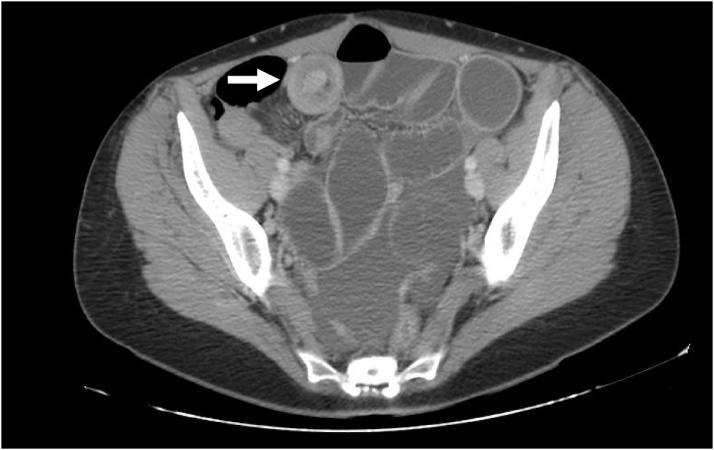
Abdominal CT evidenced a complete bowel obstruction secondary to intestinal caliber change, at the level of bowel intussusception (arrow showing the ´doughnut sign´).
